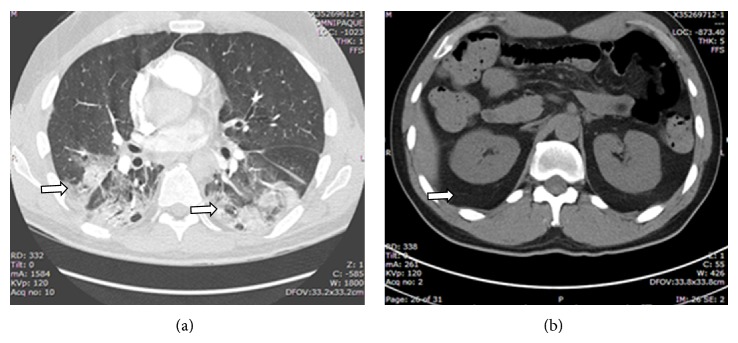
(a) CT chest/abdomen/pelvis. No evidence for pulmonary embolism. Patchy peribronchiolar consolidations are worsened in the right upper lobe, right lower lobe, and left lower lobe (see arrow). (b) No abdominal masses/hemorrhage is seen (see arrow).
